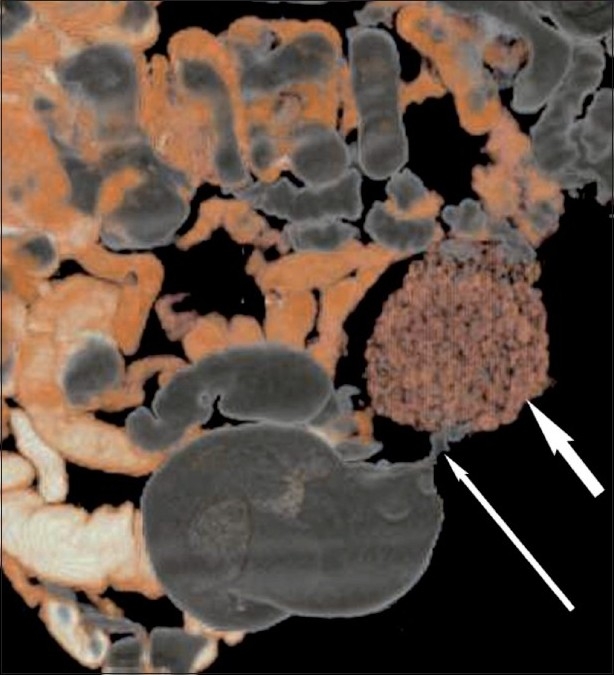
Volume-rendered CT scan showing the colonic seed bezoar (thick arrow) proximal to the sigmoid stricture (thin arrow)
